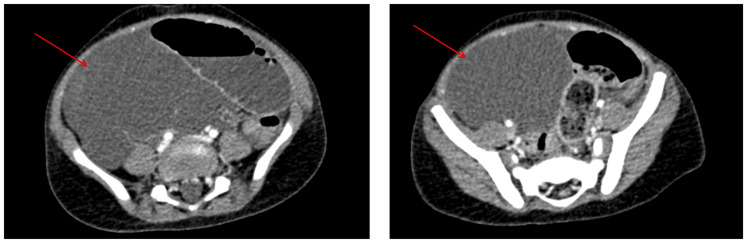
Axial slices of an abdominal CT scan showing a right-sided intra-peritoneal cystic formation displacing digestive structures to the left, without a clearly defined wall, containing fluid and without internal septations.
